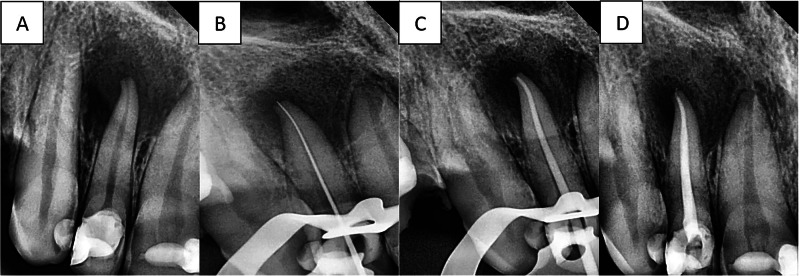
Endodontic procedure of maxillary necrotic central incisor in G2. Steps of the provided endodontic treatment in patients of G2 (A) diagnostic periapical radiograph, then (B) Working length determination, then (C) Cone Fit, and Finally (D) Final obturation.
